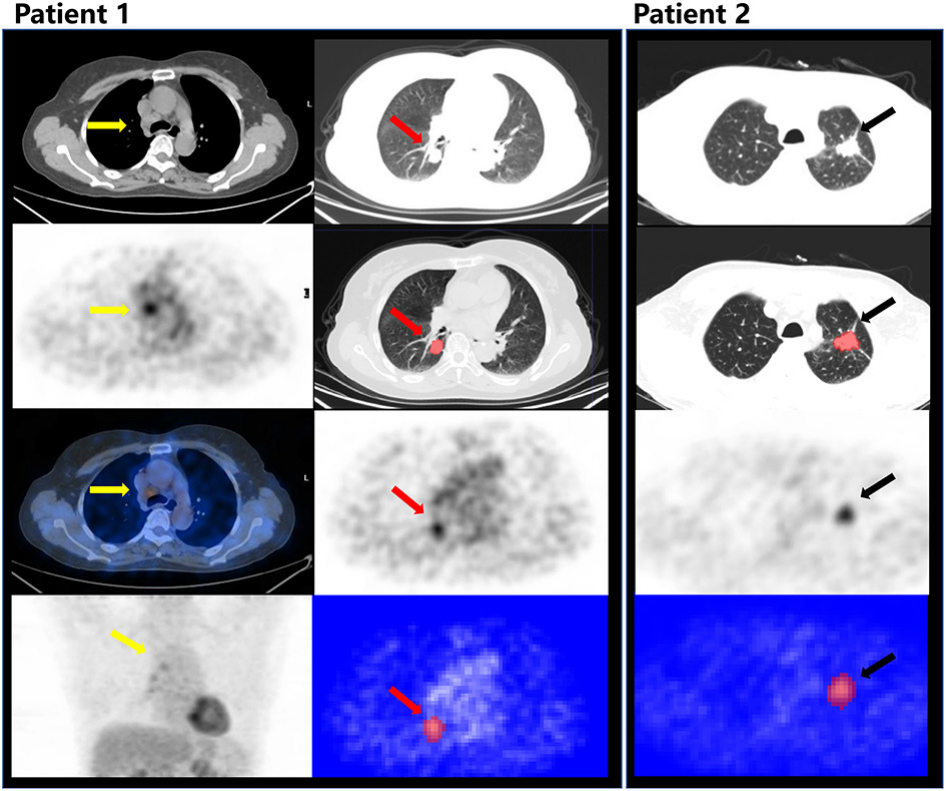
Patient 1: male, 55 years old, ADC, PET/CT showed lymph nodes in 4R (yellow arrow) with an intense FDG uptake, which was evaluated as cN2, predicted as N0 by the RM, and confirmed as pN0 after radical resection. The red arrow indicates the tumor in the right lower lobe (CT, PET) and the segmentation on ITK-SNAP. Patient 2: male, 66 years old, ADC, evaluated as cN0, but predicted as N+ by the RM, and confirmed as pN1 after radical resection. The black arrow indicates the primary lesion in the left upper lobe (CT, PET) and the segmentation on ITK-SNAP.
